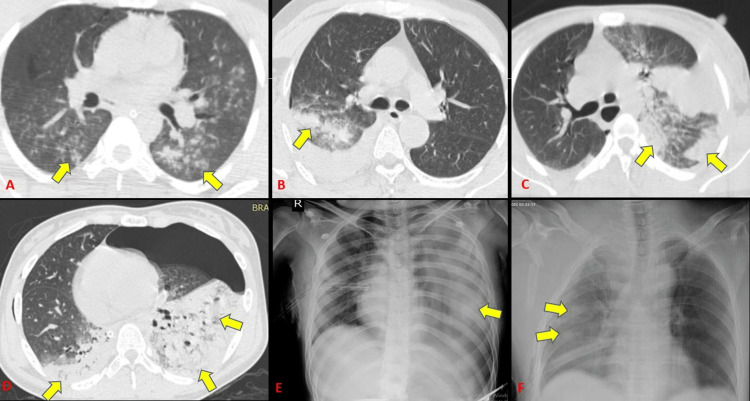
Varied presentation of lung contusions seen on CT and radiographs.
